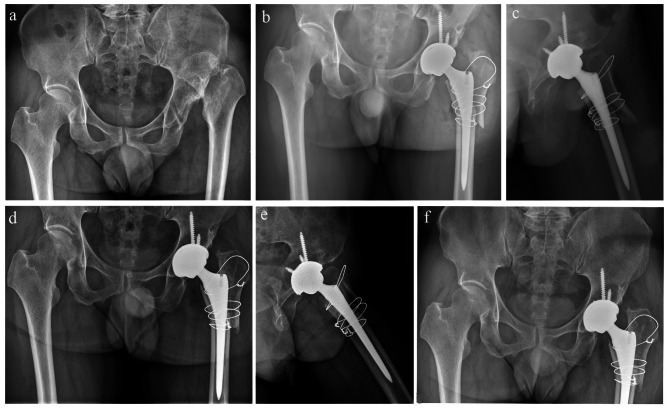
a preoperative anteroposterior radiography of the pelvis; b, c X-ray after reconstruction of proximal left femur + THA; d, e X-ray reexamination 6 months after surgery showed that the proximal femur reconstruction had basically healed. f at the 1-year postoperative follow-up, the prosthesis was in good position
